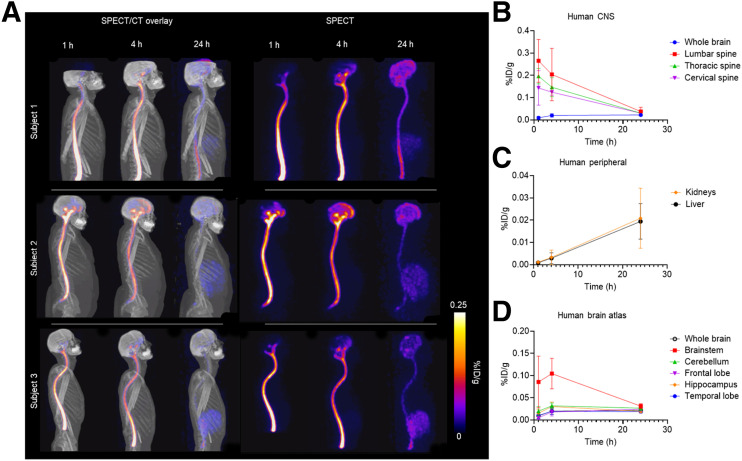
SPECT imaging of [^99m^Tc]Tc-MAG3-tofersen in healthy human volunteers. (A) Maximum-intensity-projection images showing distribution of [^99m^Tc]Tc-MAG3-tofersen in humans (*n* = 3) 1, 4, and 24 h after intrathecal injection. Time–activity curves derived from SPECT data measuring activity in ROIs in CNS (lumbar, thoracic, and cervical spine ROIs include both cord and proximal CSF) (B), kidneys and liver (C), and brain atlas (D).
